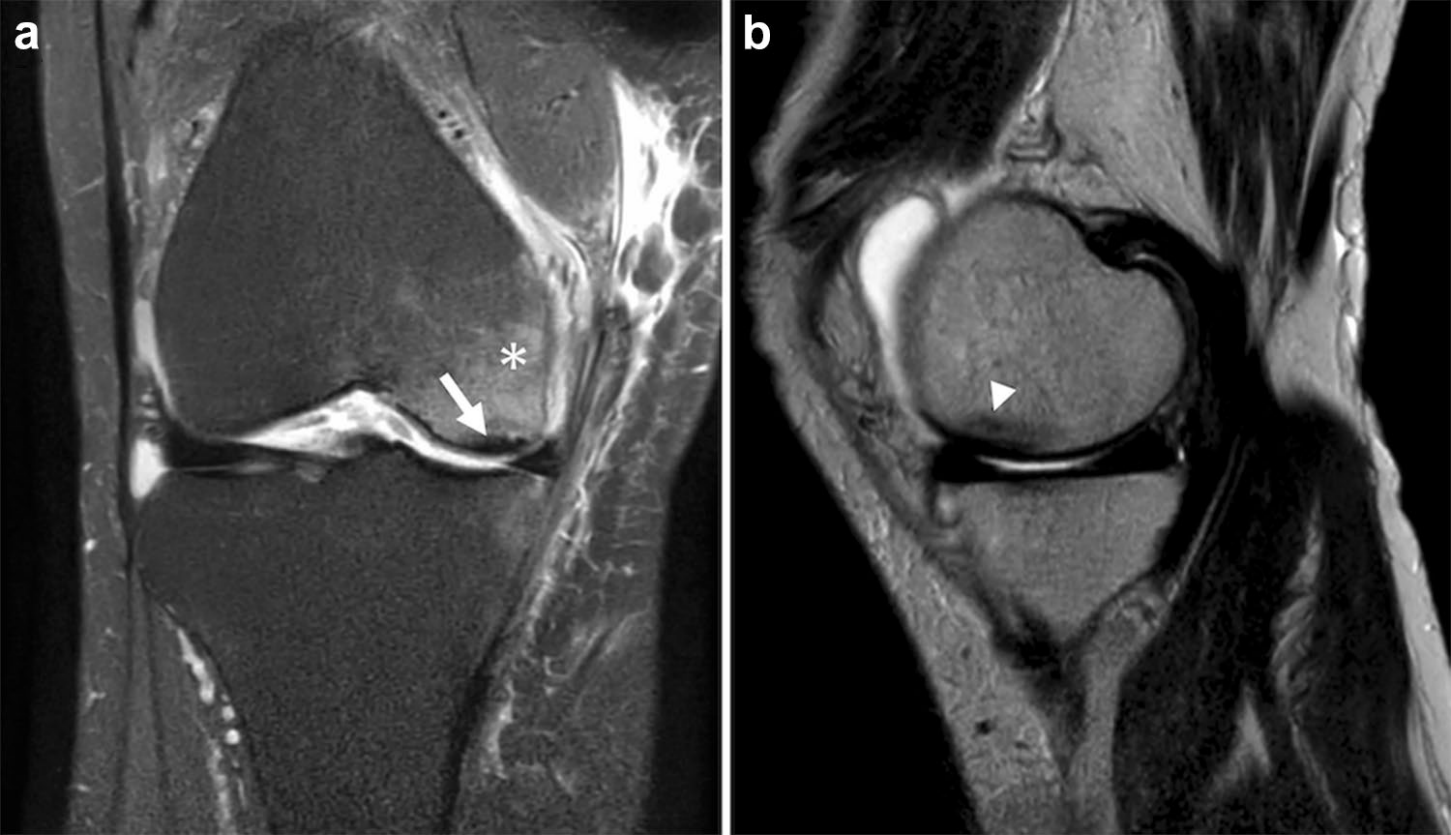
MRI of a 73-year-old female patient with SIFK in the medial femoral condyle of the right knee. a Coronal FS-PDWI shows extensive bone marrow edema-like signal intensity over the medial femoral condyle (asterisk) and a subchondral area of low signal intensity (arrow), which is integrated with the subchondral plate and resembles a thickened subchondral plate. Note the medial meniscal extrusion which is an associated finding. b A sagittal T2-weighted image (T2WI) showing a subtle hypointense line (arrowhead) above the subchondral area of low signal intensity
